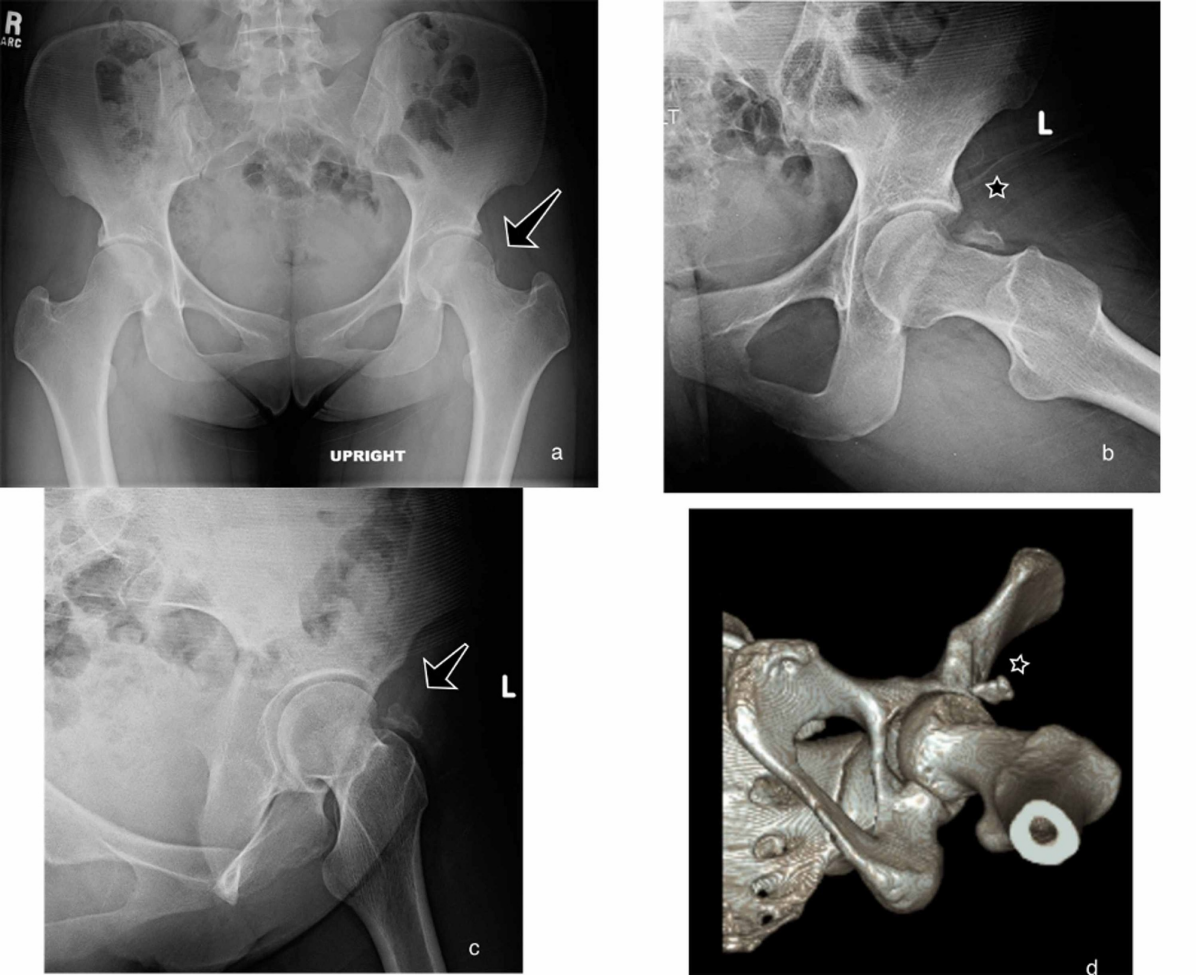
Case 1: Preoperative Imaging (a) AP pelvis with HO seen on the left hip (arrow). (b) Modified Dunn view showing grade 1 HO in the hip capsule (star). (c) False-profile view showing HO (arrow). (d) 3D reconstruction CT-image of the left hip demonstrating HO (star).
